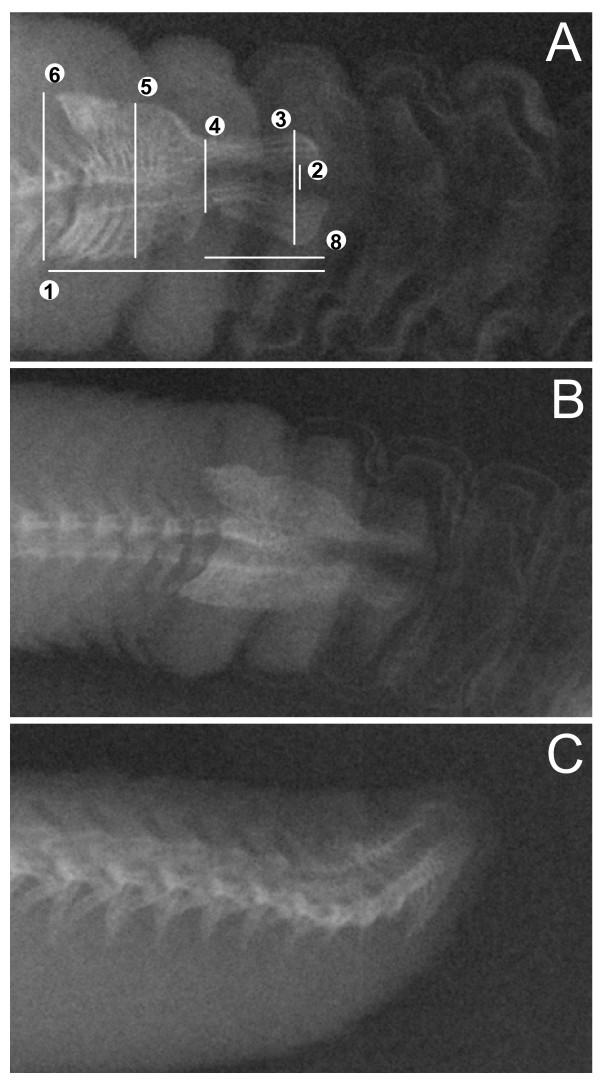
Radiographs of representative rattlesnake styles. (A) *Crotalus mitchellii *(UTA R-51444), showing linear measurements used in Evolutionary Principal Components Analysis (EPCA). Measurement 7 (not shown) is the transverse width of the style at its widest point. (B) *Crotalus ruber *(UTA R-7235). (C) *Crotalus catalinensis *(UTA R-32129).
